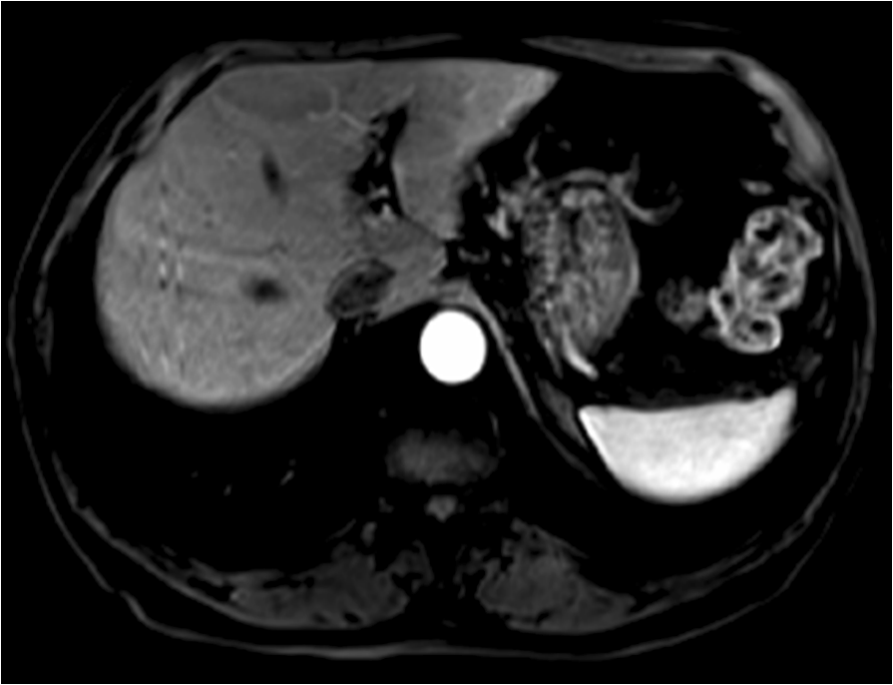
Axial three-dimensional arterial phase gradient echo (GRE) T1-weighted images demonstrating mild homogeneous enhancement of the mass.
